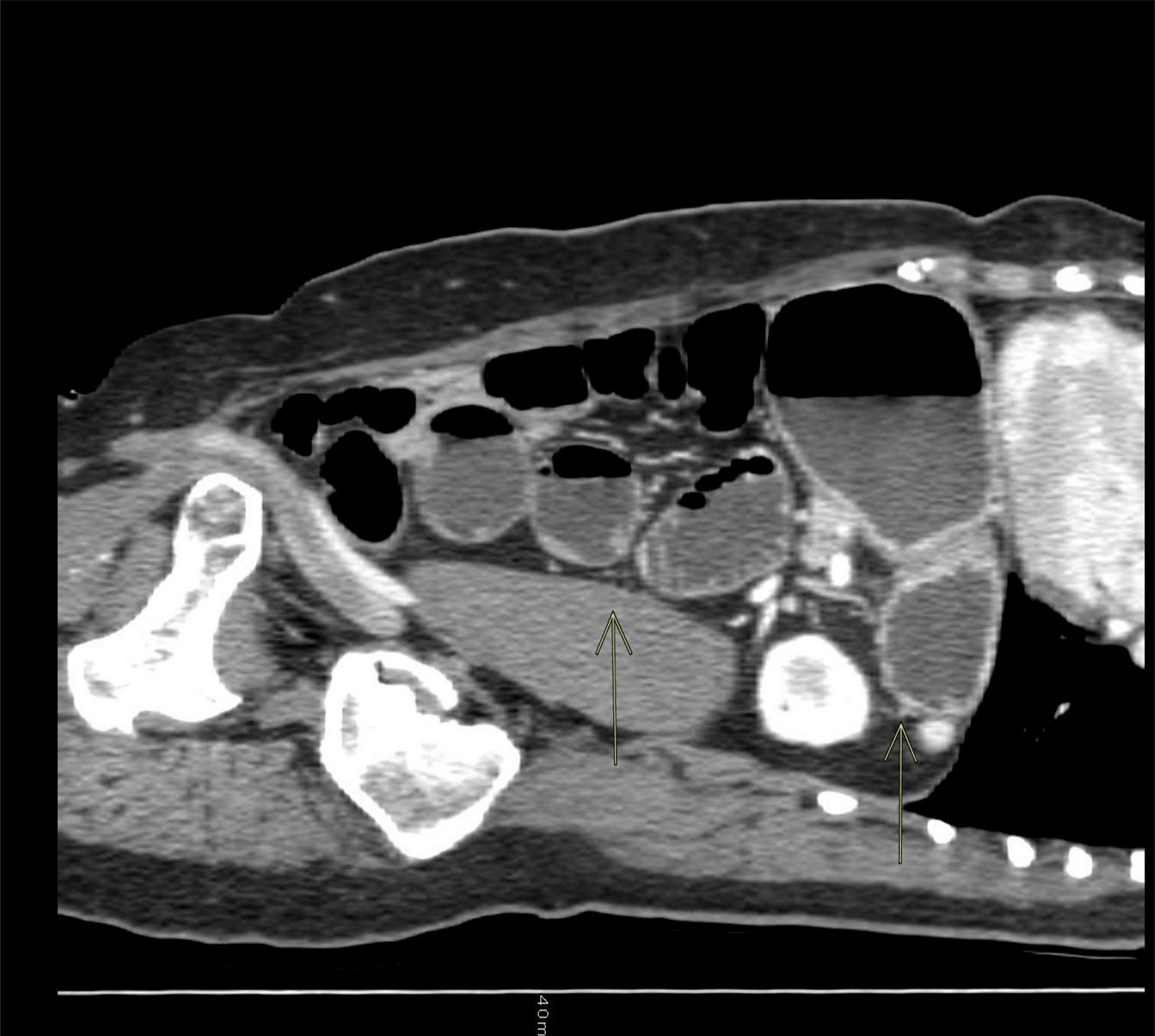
Sagittal CT scan of the abdomen: the arrows show a dilated stomach and ileal levels.
